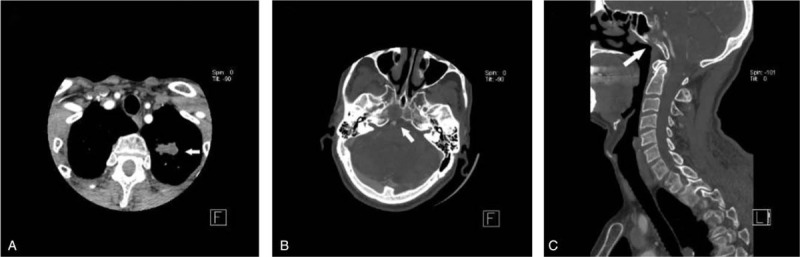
Axial (A) CTA image demonstrating a 1.7 cm pulmonary nodule in upper lobe of the left lung (white arrow). This incidental finding was initially mentioned on neck CTA. Axial (B) and sagittal (C) CTA images demonstrating a bony destruction of sphenoid bone (white arrow), which was, inversely, not initially reported.
